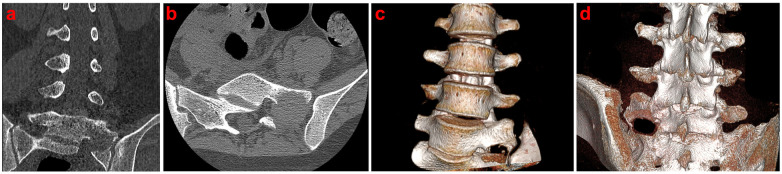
Postoperative sagittal (a) and axial (b) CT scans of the lumbar region, as well as three-dimensional computed tomography (CT) scans of the lumbar spine (c,d), show that the artificial joint has been removed.
